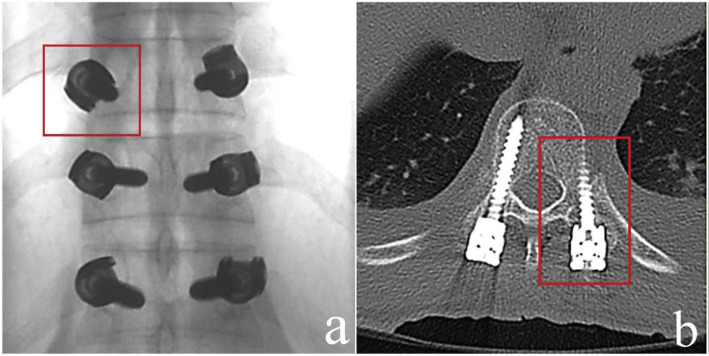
a) Intra-operative fluoroscopy image showing the tip of the left thoracic level 10 pedicle screw being overlapped by the screw head; b) Postoperative axial CT image showing a major lateral beach of the pedicle.
